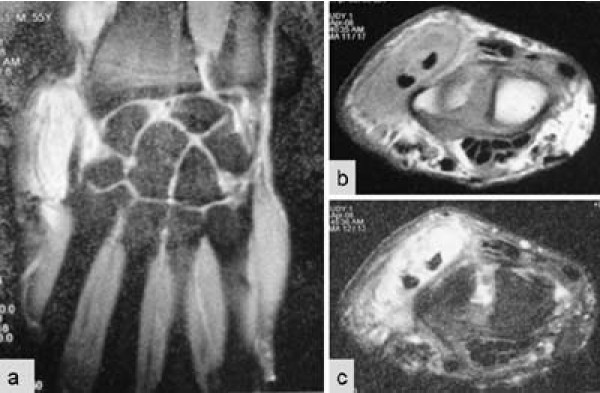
Magnetic resonance imaging of the wrist. (a) Coronal section proton density fat saturation; (b) axial section T1 fat saturation images showing hypointense signals on dorsolateral aspect of the wrist; (c) axial section T2 fat saturation images showing hyperintense signals on the radial side of the wrist joint surrounding the tendon.
